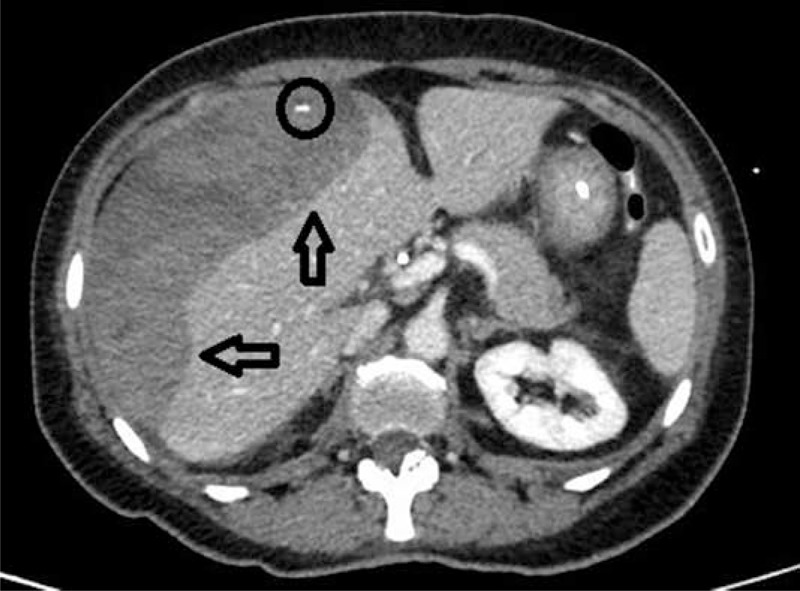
CT scan of middle abdomen showing a subcapsular hepatic hematoma on the surface of the right lobe of liver (hypodense area indicated by the arrows) in which is present a source of active bleeding (circle). CT = computed tomography.
